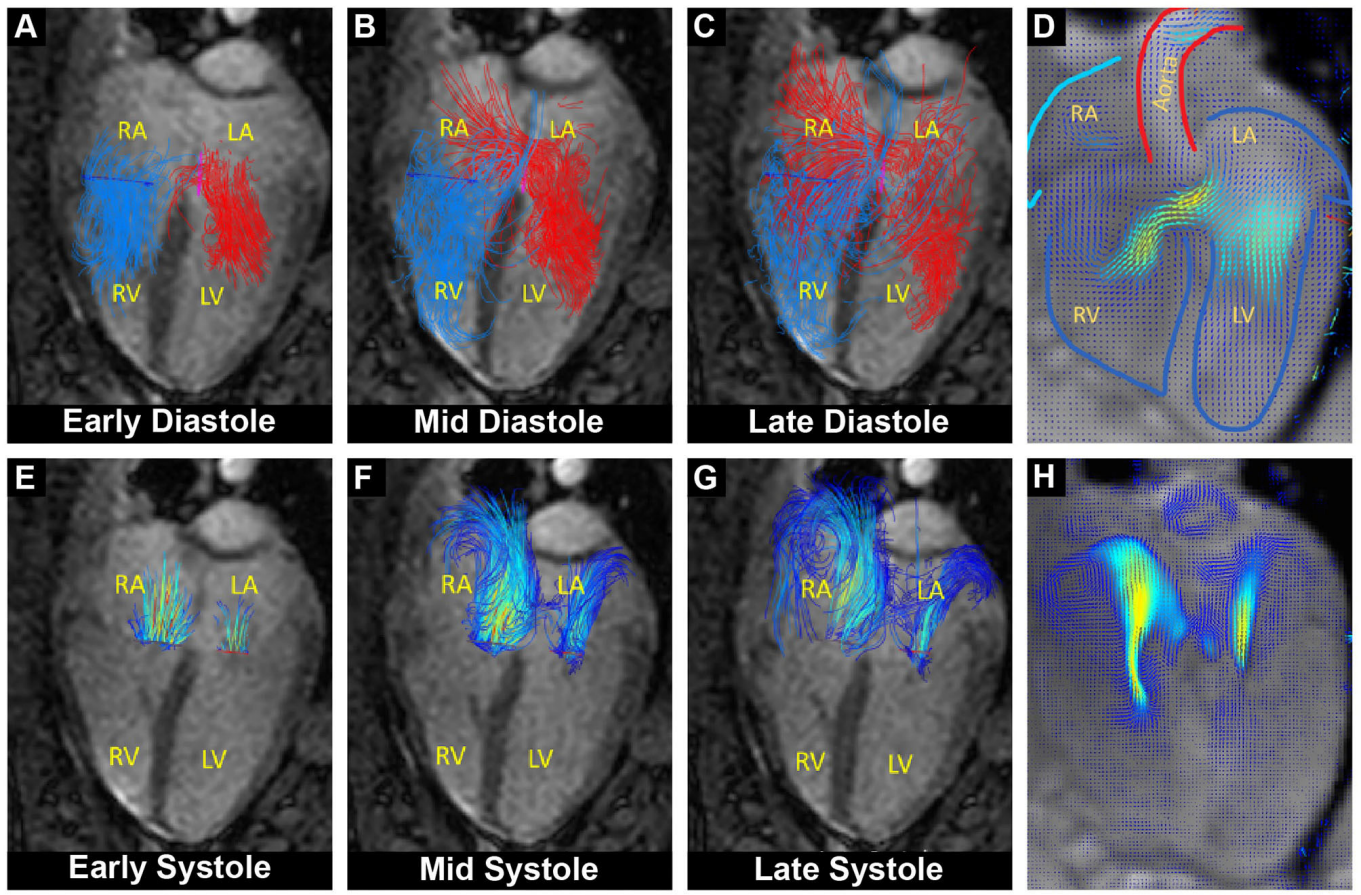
Overlay of cardiac functional MRI and pathline visualization of blood flow during the cardiac cycle. RA, right atrium; LA, left atrium; RV, right ventricle; LV, left ventricle. (A–C) Pathline visualization of blood flow originating from the left (red) and from the right (blue) atrium, showing left-to-right flow across the atrial septum during diastole (L-R shunt). (D) Velocity field visualization focused on the diastolic shunt across the atrial septum. (E–G) Pathline visualization of regurgitant blood flow through the closed mitral and tricuspid valve during systole. (H) Velocity field visualization focused on the systolic mitral and tricuspid regurgitant flow.
